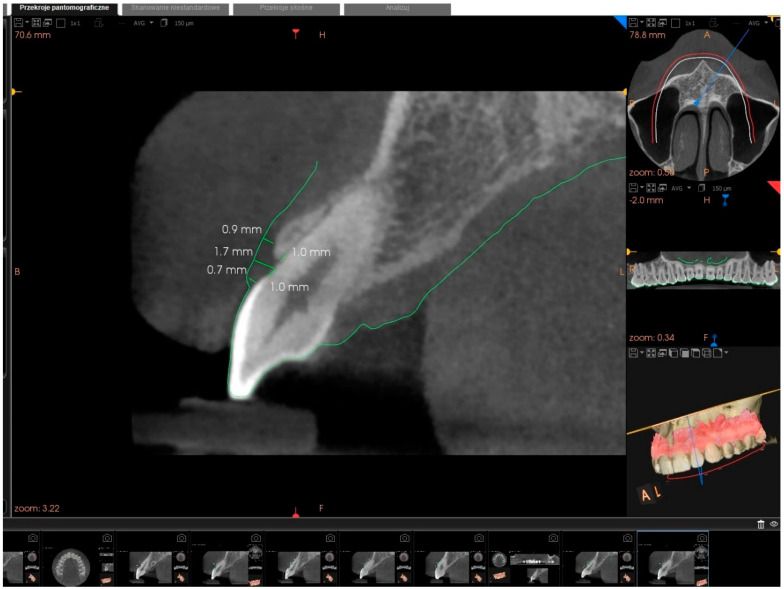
Cone-beam computed tomography, intraoral scanning by computer-aided design technology along with prosthetic-driven implant planning software for FGT, SGT, and CGT measurement.
